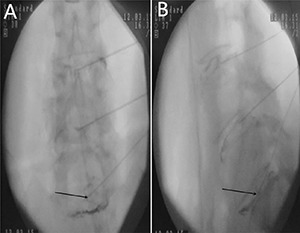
Provocation discography for a 54 years old woman, who had sufferedfrom low back pain (LBP) for more than 2 years The results shows the ruptured intervertebral disc (L5-S1), leakage of the contrast medium, concordant pain (7 point on the numeric rating scale) was induced according to the VAS score. (A) is the frontal X-ray of the provocation discography. (B) indicates the lateral X-ray of the provocative discography.
